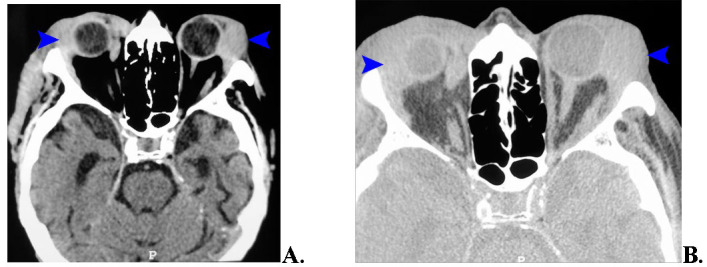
A. The axial image shows bilateral, homogeneous, relatively well-defined formations located superolateral to the eyeballs (blue arrows). B. Axial CT brain image - bone window - the tumor formations (blue arrow) do not cause erosion of the orbital walls
